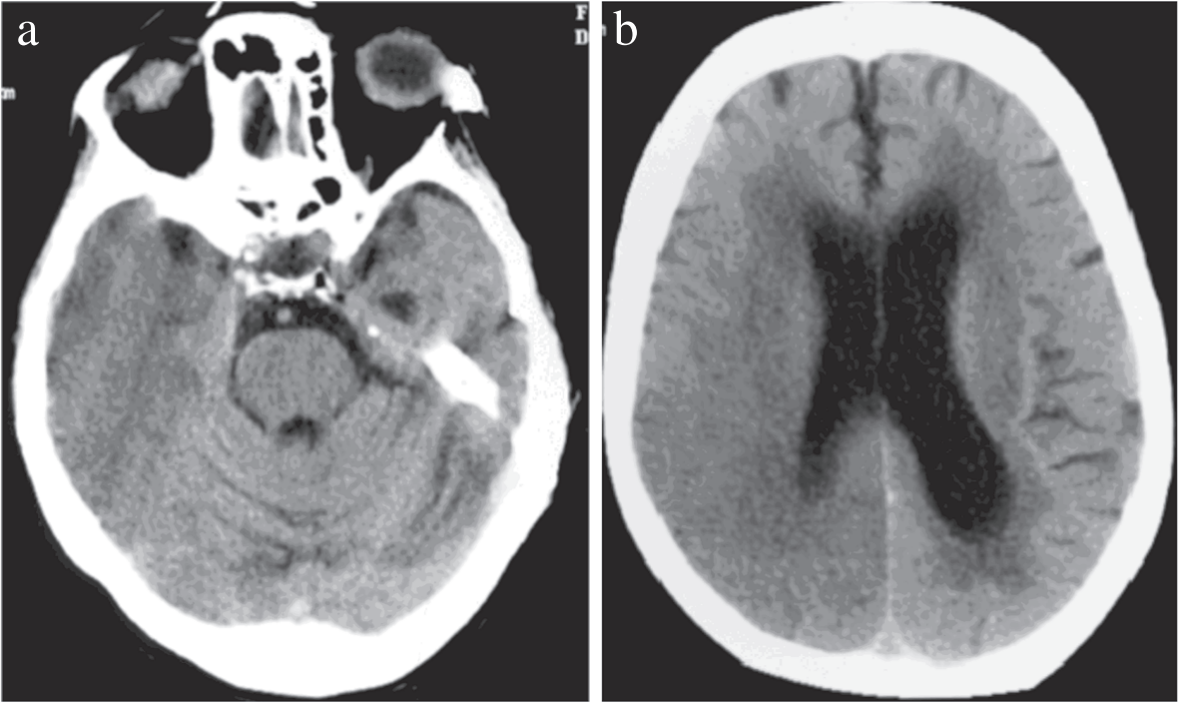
86 year old female in hypoglycaemic coma, computed tomography on Day 14 after onset of hypoglycaemia. There is a large area of low attenuation involving grey and white matter in the right temporal and parietal lobes. Differential diagnosis for this imaging appearance would be acute right middle and posterior cerebral artery territory infarction.
